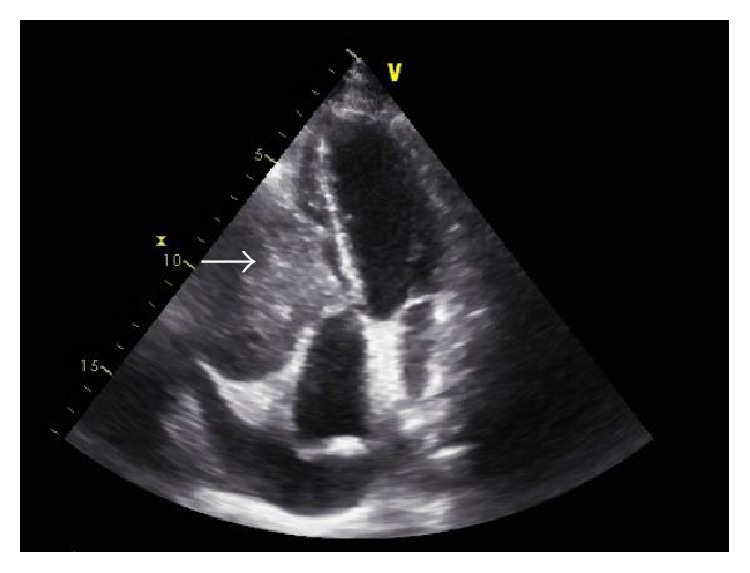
2D echocardiogram of the heart in apical four chamber view showing a right ventricular mass (arrow) arising from the free wall.
